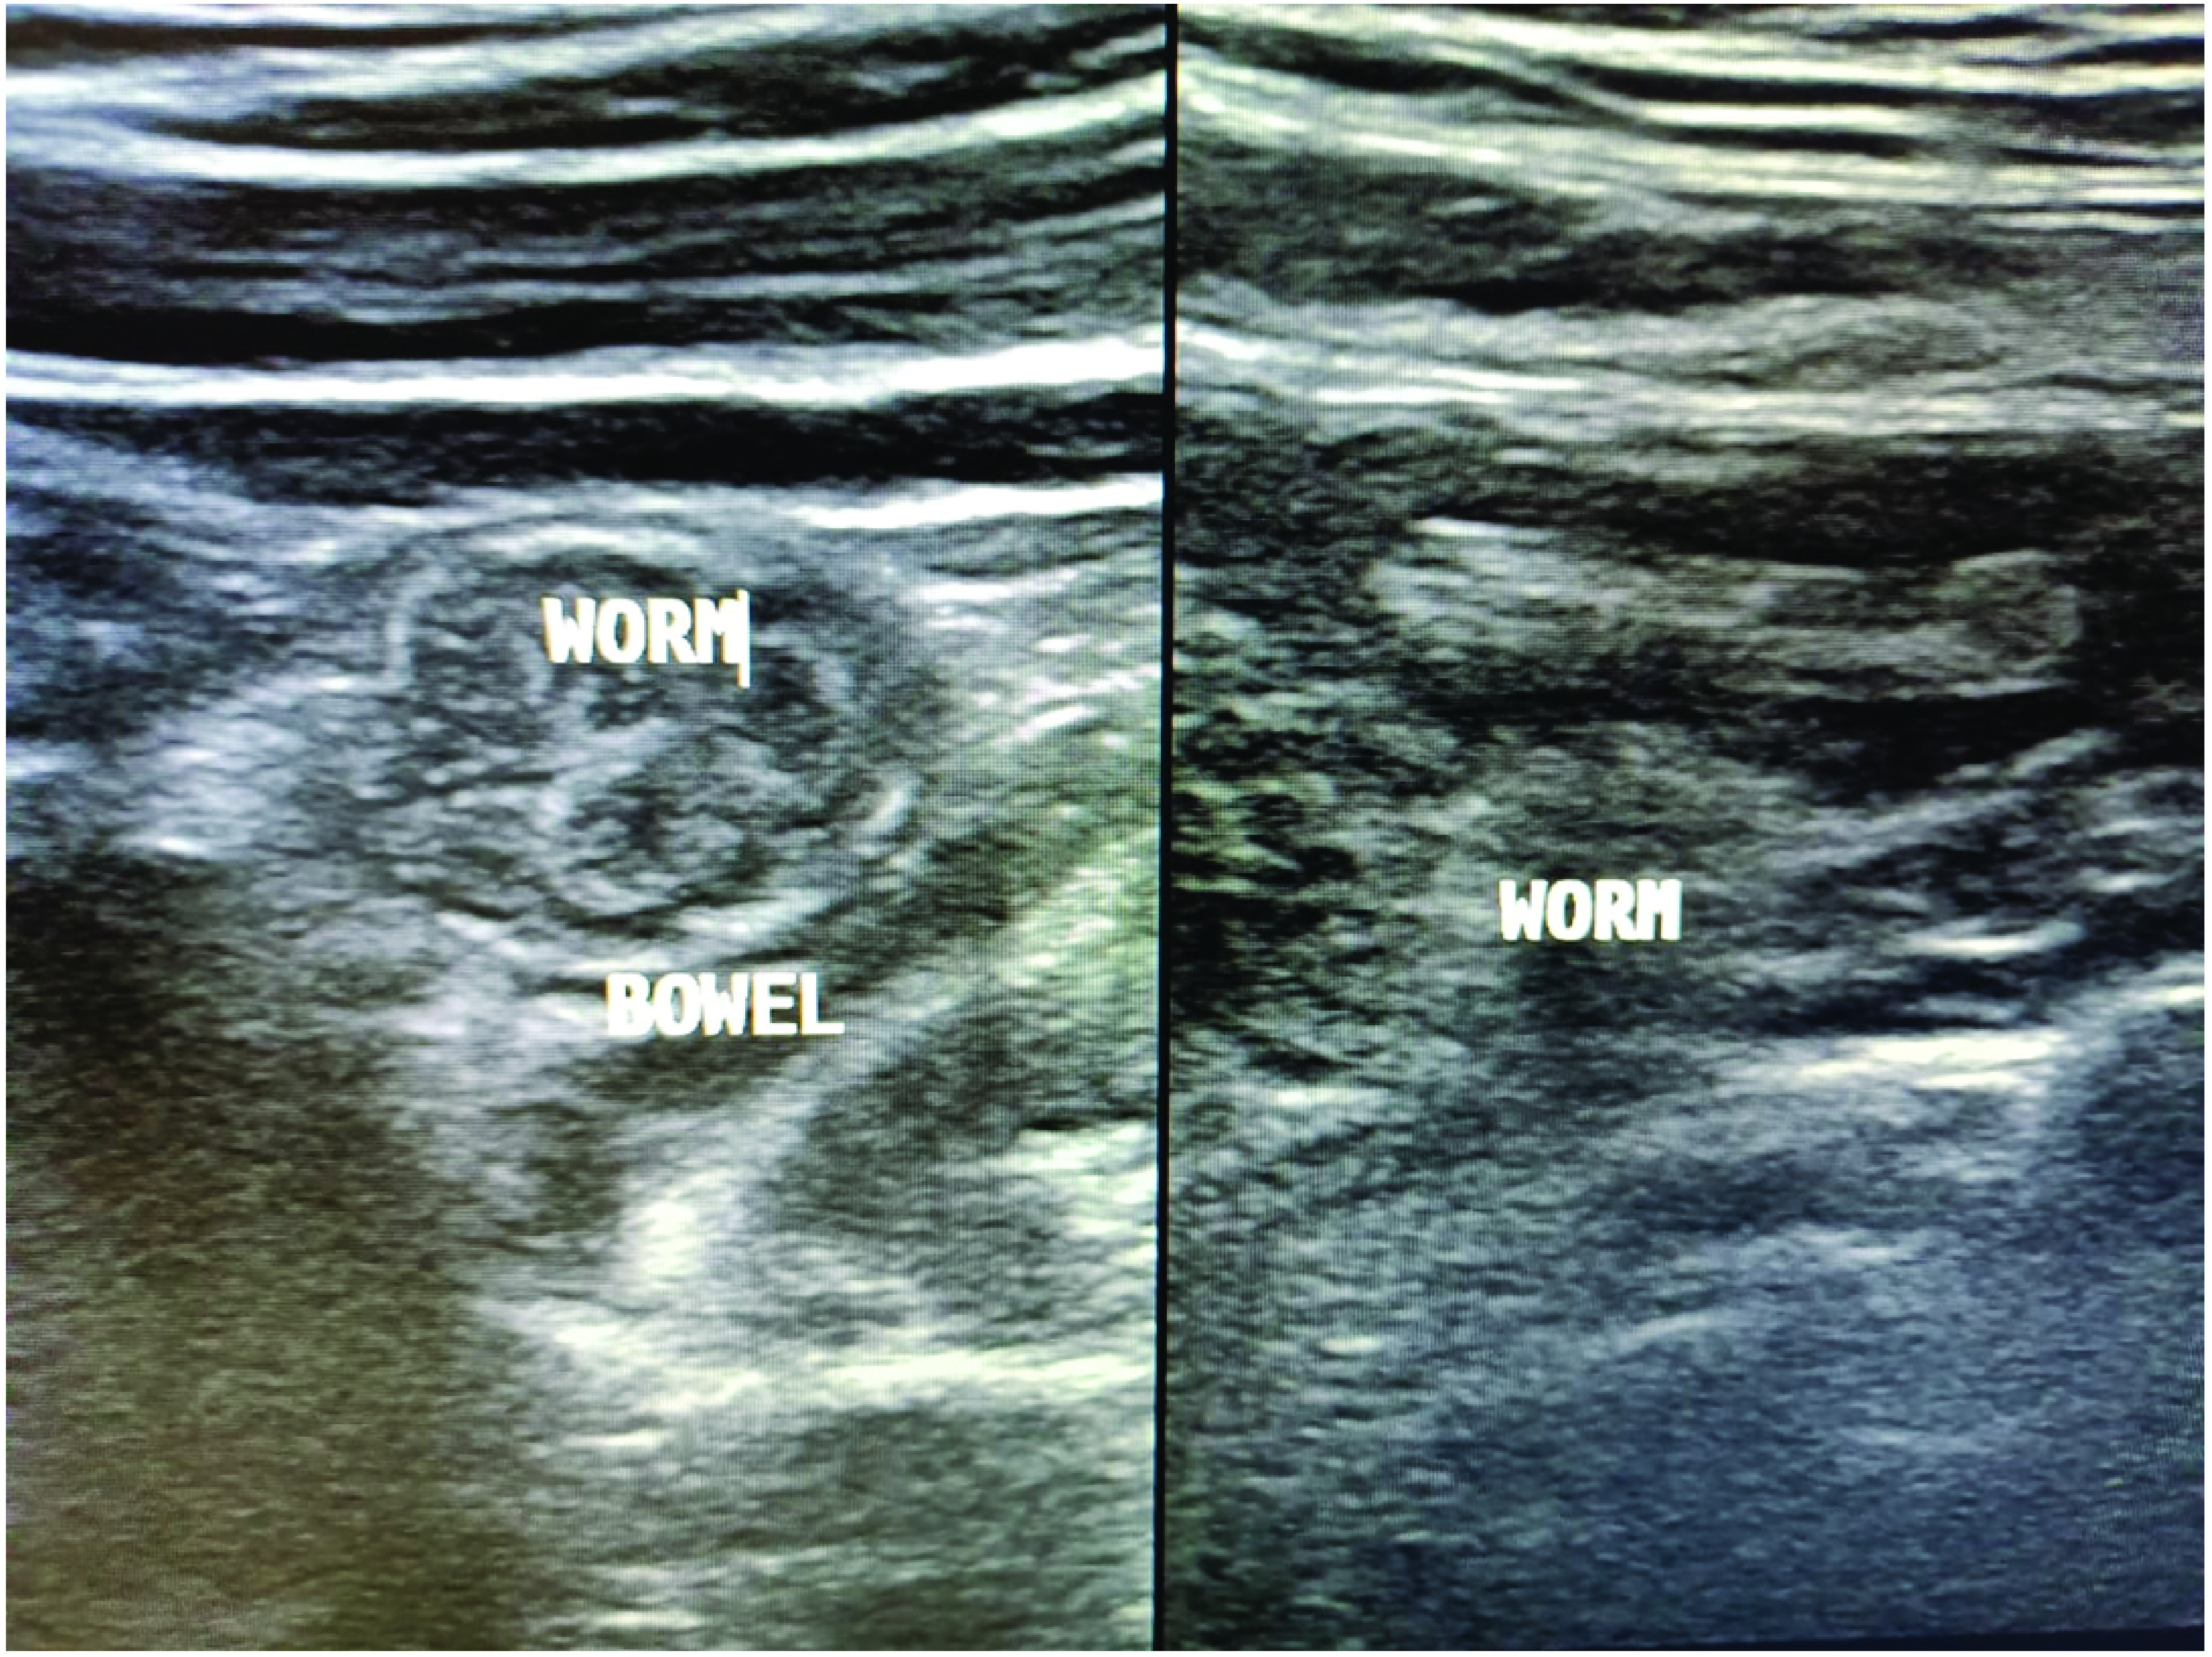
Abdominal ultrasound showing multiple tubular echogenic structures resembling *Ascaris lumbricoides*.
